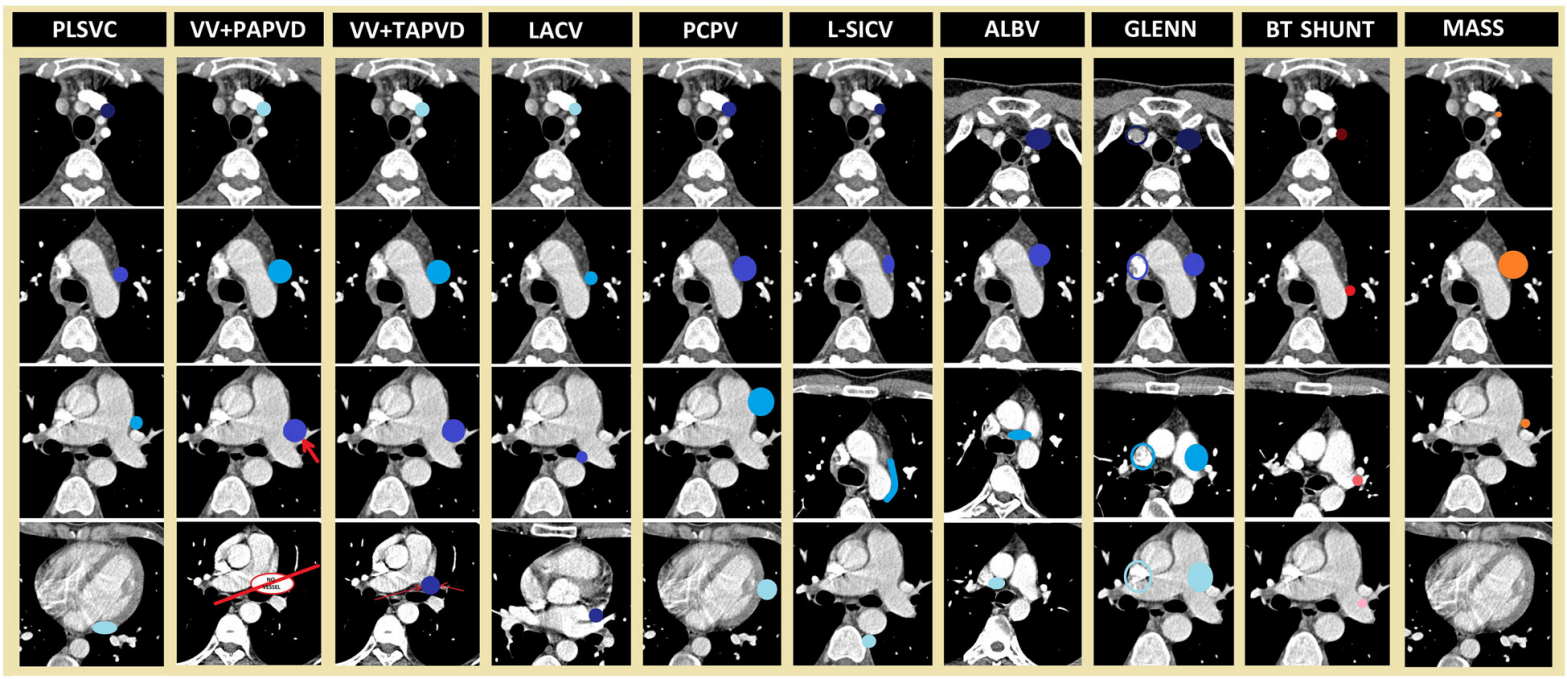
A comprehensive summary of differential diagnoses of PLSVC. The course of the most frequent PLSVC variation (right atrial drainage via coronary sinus) and the courses of possible differential diagnoses are shown as columns with axial CT images from superior to inferior. For depicting the expected flow direction of vascular structures, the upstream zones are marked with darker shades, whereas the downstream zones with lighter shades. Red arrows represent pulmonary veins. Blue circles are used to depict systemic venous structures, while red circles are used to show the BT shunt, which is an interarterial structure. Orange circles represent the mass. In the column of Glenn shunt, the course of PLSVC is shown with solid blue circles while the course of RSVC is shown with hollow blue circles. Please note that (1) in the second row of the figure, all differential diagnoses of PLSVC are observed to be in a similar location in the mediastinum. (2) While two vascular structures are seen in front of the left main bronchus in the presence of PLSVC, no vascular structure is seen in this area in the presence of VV with PAPVD. (3) Expected flow directions fo VV and LACV are caudocranial, unlike other vascular structures shown in the figure. Also, LACV is located in the posterior of the pulmonary artery, unlike PLSVC, which is located anterior to the pulmonary artery
